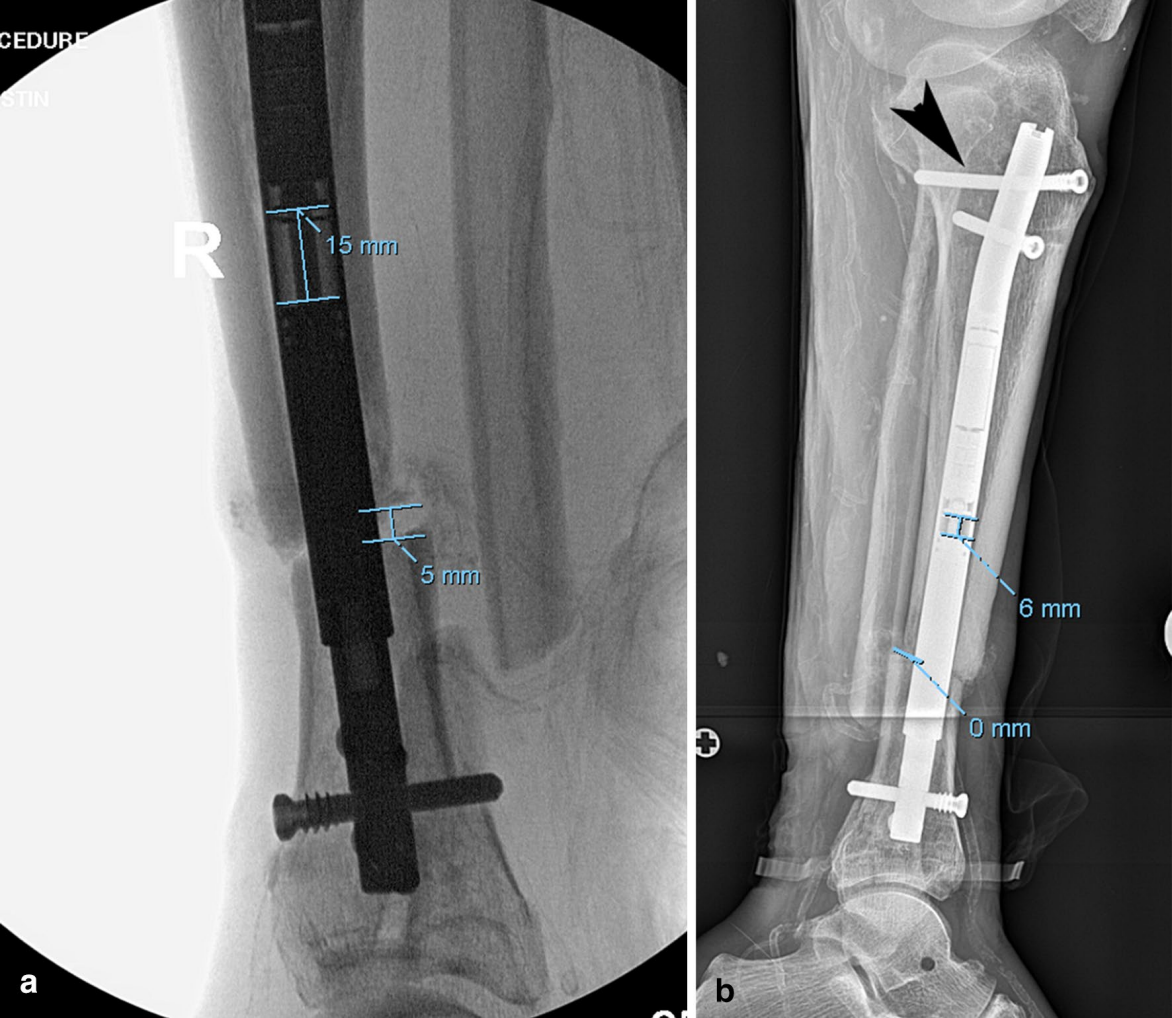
a This radiograph is from case #6. The nail is predistracted 15 mm, and there is a bone gap of 5 mm at the nonunion site. b The nail has shortened by 9 mm with a residual 6 mm of potential space for additional compression. There is no space at the nonunion site. The proximal bolt (black arrow) is bending
